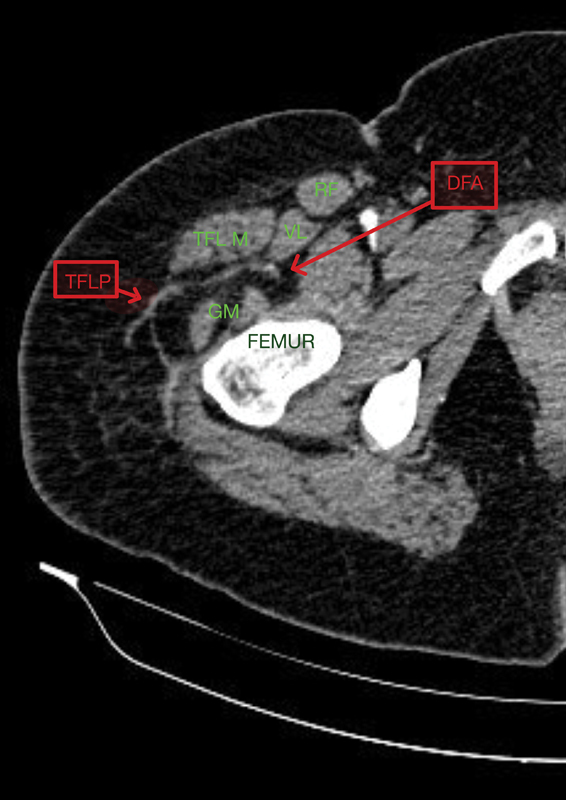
CT angiogram image of TFL perforator (TFLP) originating from the deep femoral artery (DFA) and emerging between the TFL muscle and gluteus medius (GM) muscle. RF, rectus femoris; TFL, tensor fascia lata; VL, vastus lateralis.
